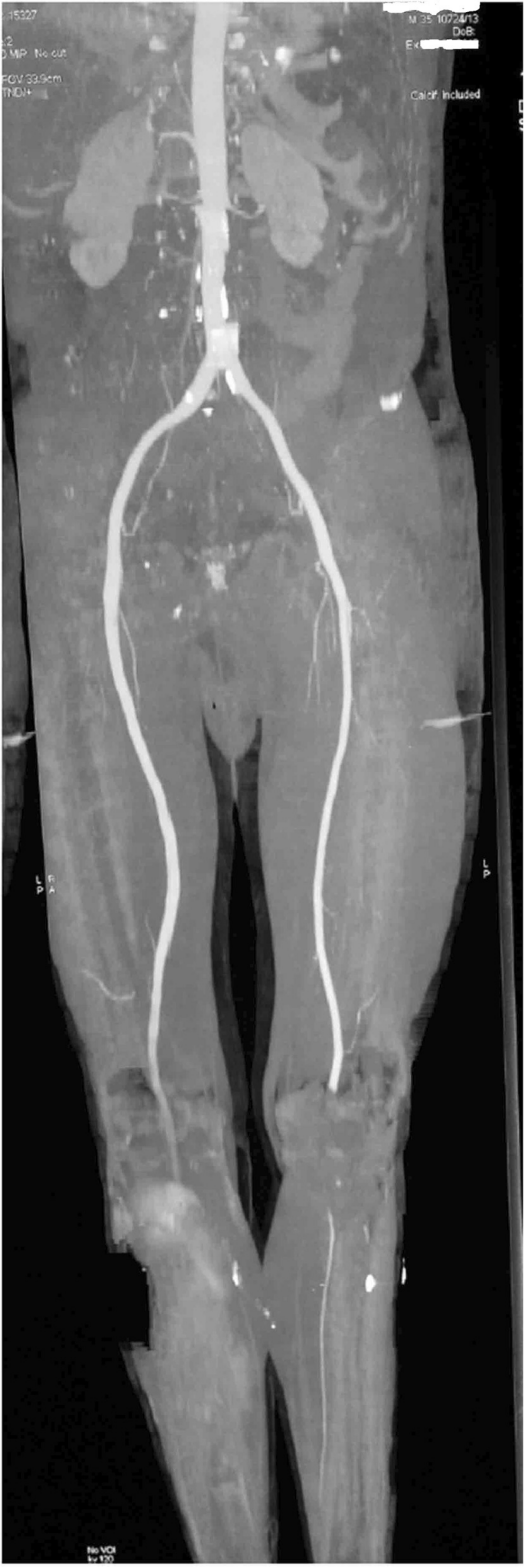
CT angiography of the lower limbs showing. Right; poor patency of the operated arterial axis, and Left; a change in contrast at the beginning of the popliteal artery.
